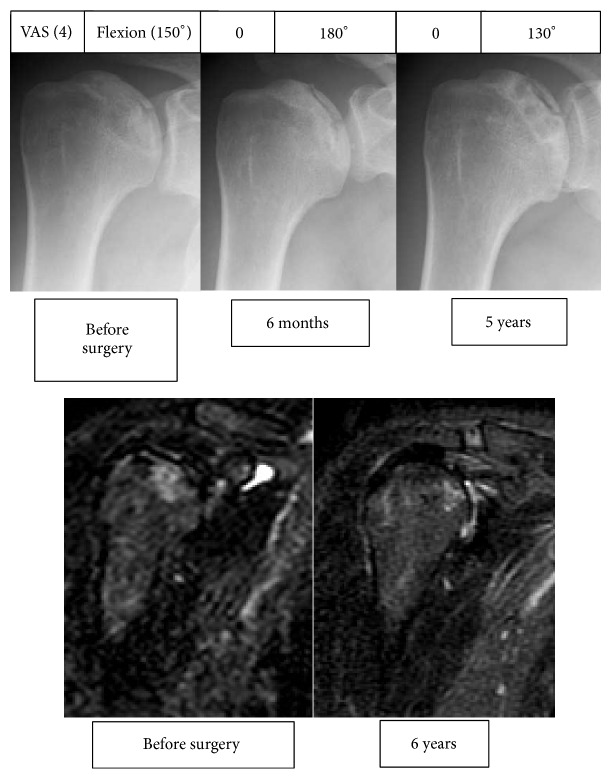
Radiographic and magnetic resonance images of the right shoulder in Case 1. The box on top of the radiographic images shows the visual analogue scale on the left and the range of flexion on the right.
